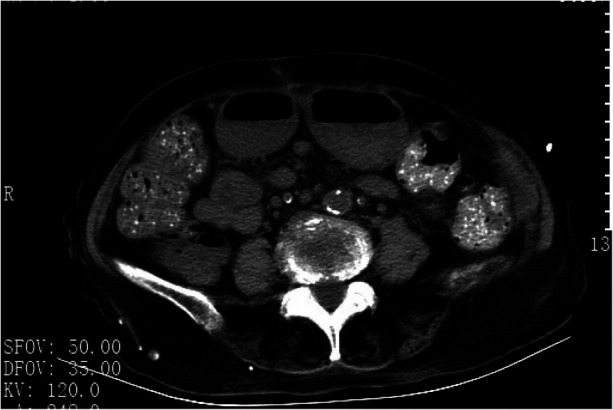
Abdominal CT image obtained two months after percutaneous abscess drainage.
